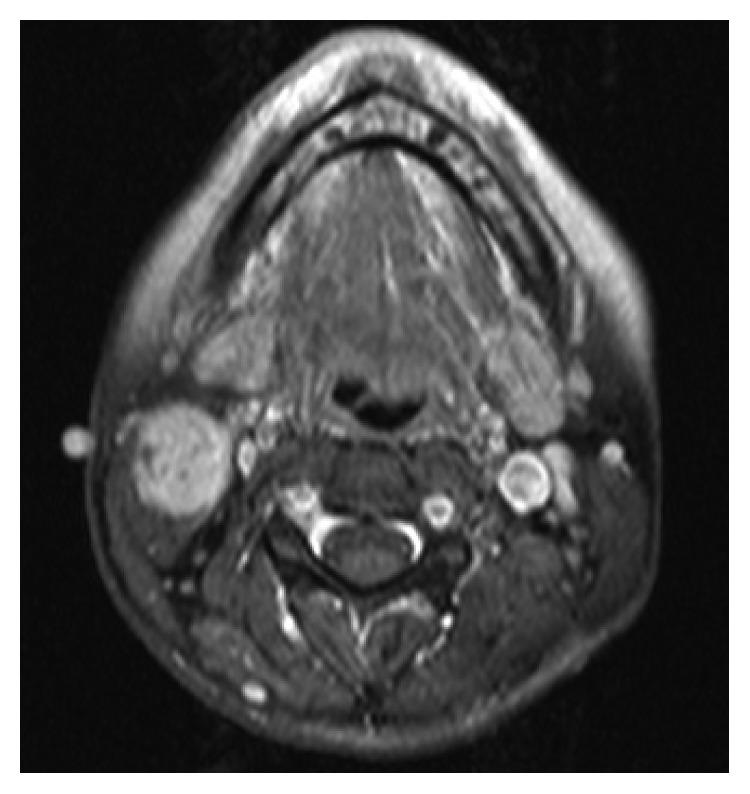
Axial T1-weighted fast spin-echo (T1FSE) MRI showing an enhancing mass indicative of local tumor recurrence following surgery.
